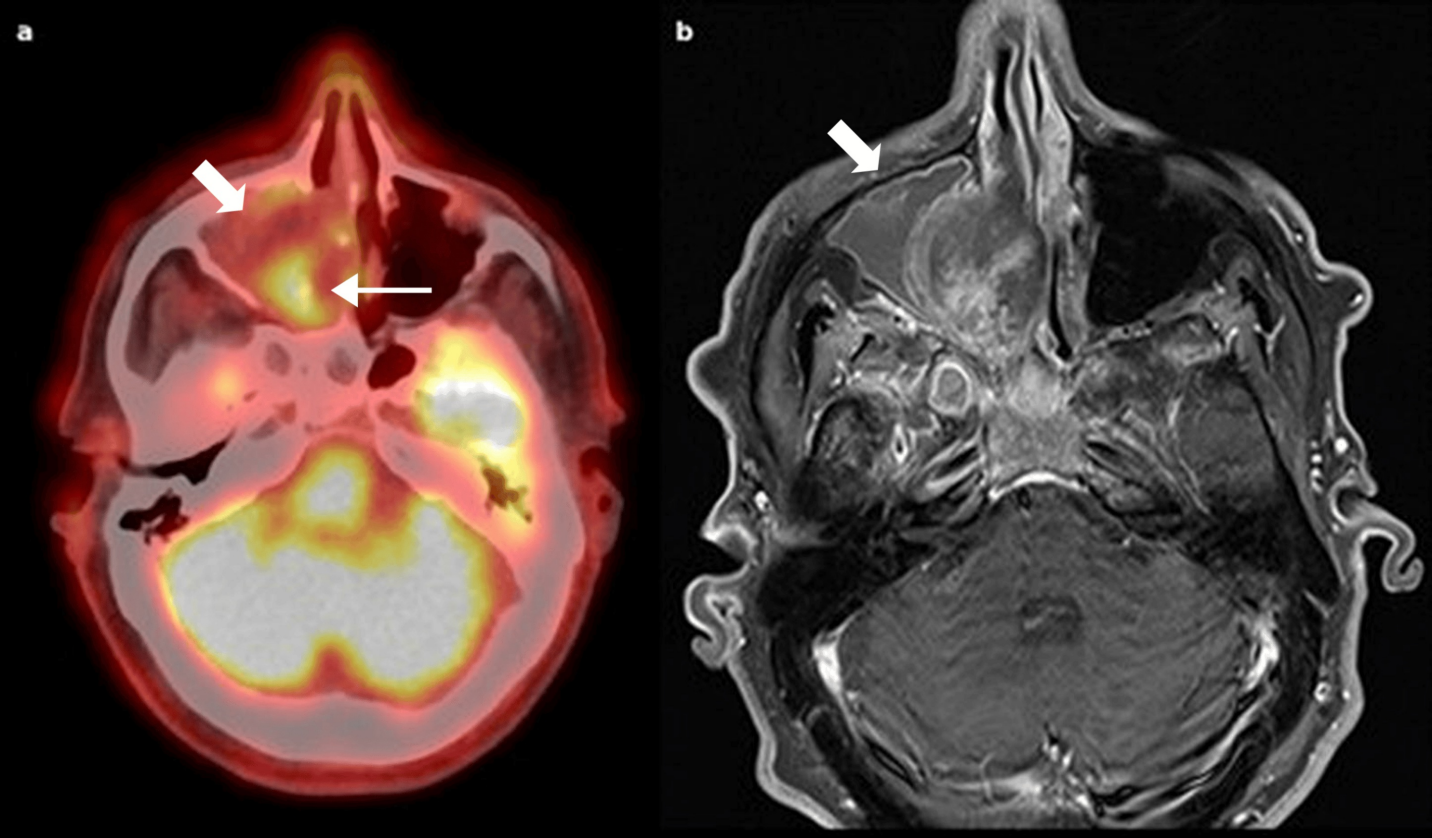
Axial fused PET-CT and corresponding contrast-enhanced T1-weighted MRI Axial fused PET-CT image (a) and corresponding contrast-enhanced T1-weighted MRI (b) at the same anatomical level. Note the absence of fluorodeoxyglucose (FDG) uptake in the right maxillary sinus, consistent with fluid content (thick arrow). Thin arrow representing the FDG uptake into the inferior ethmoidal part of the mass.
